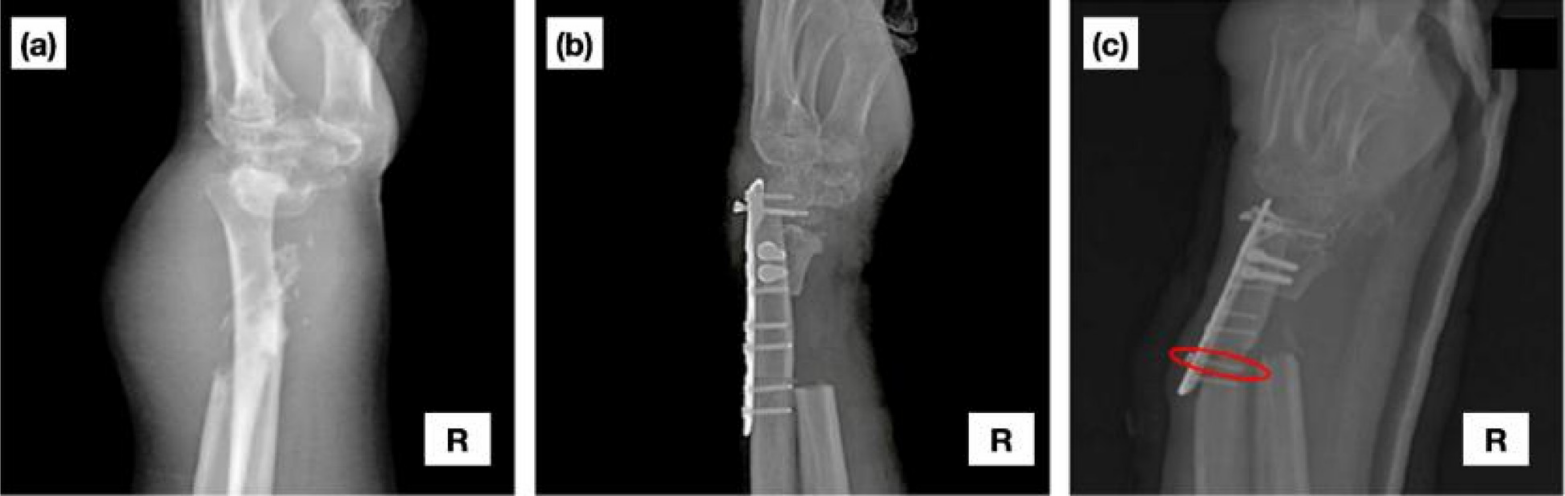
Radiographic comparison of surgical intervention in a 60-year-old man's right hand (a) before and (b) following en bloc resection surgery. (c) Bone deformation and screw loosening from its position at the second bottom of the locking distal radius plate are indicated by a red ellipse.^27^
